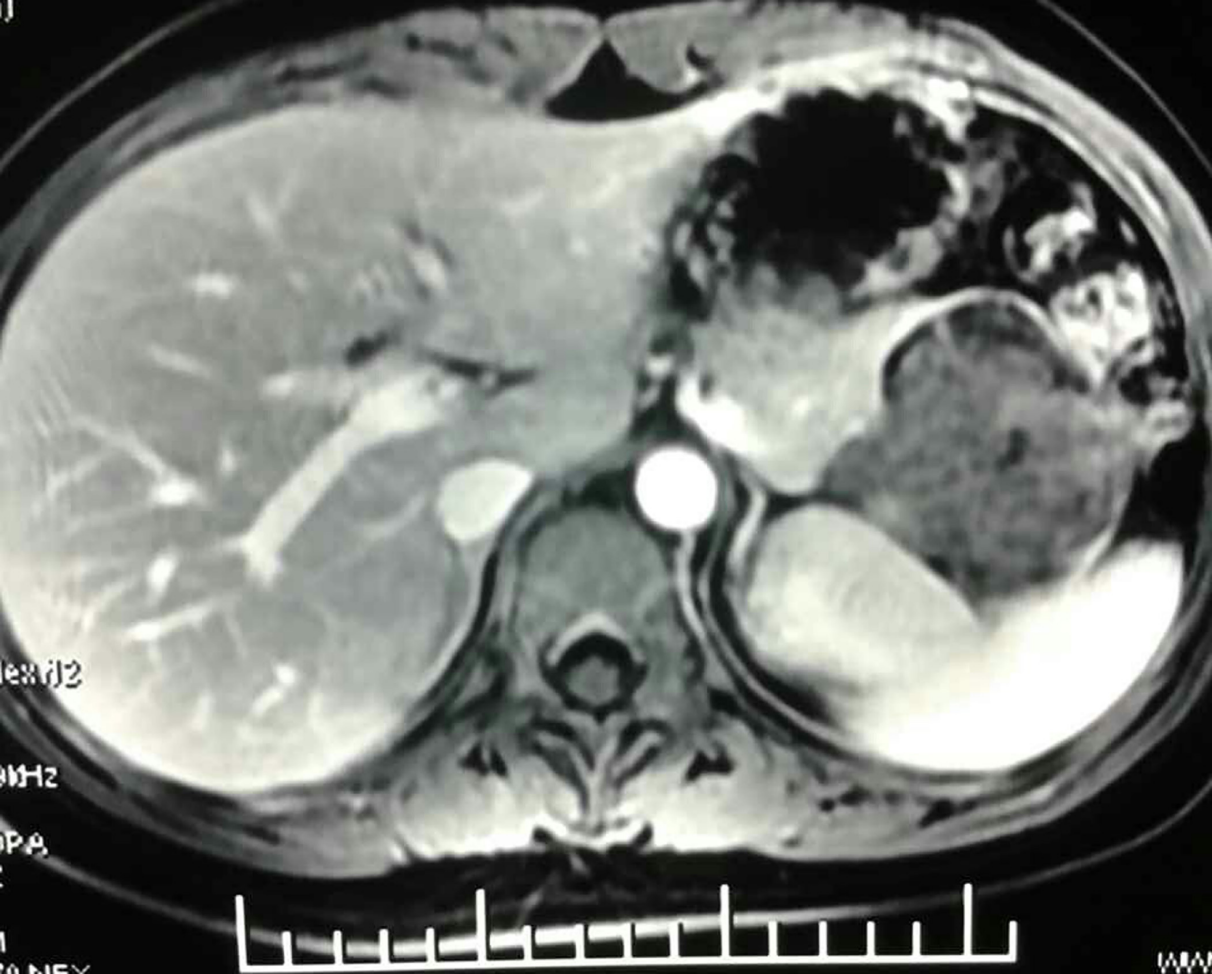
CT examination of SPT shows a well-encapsulated, heterogeneous cystic and solid pancreatic mass.
